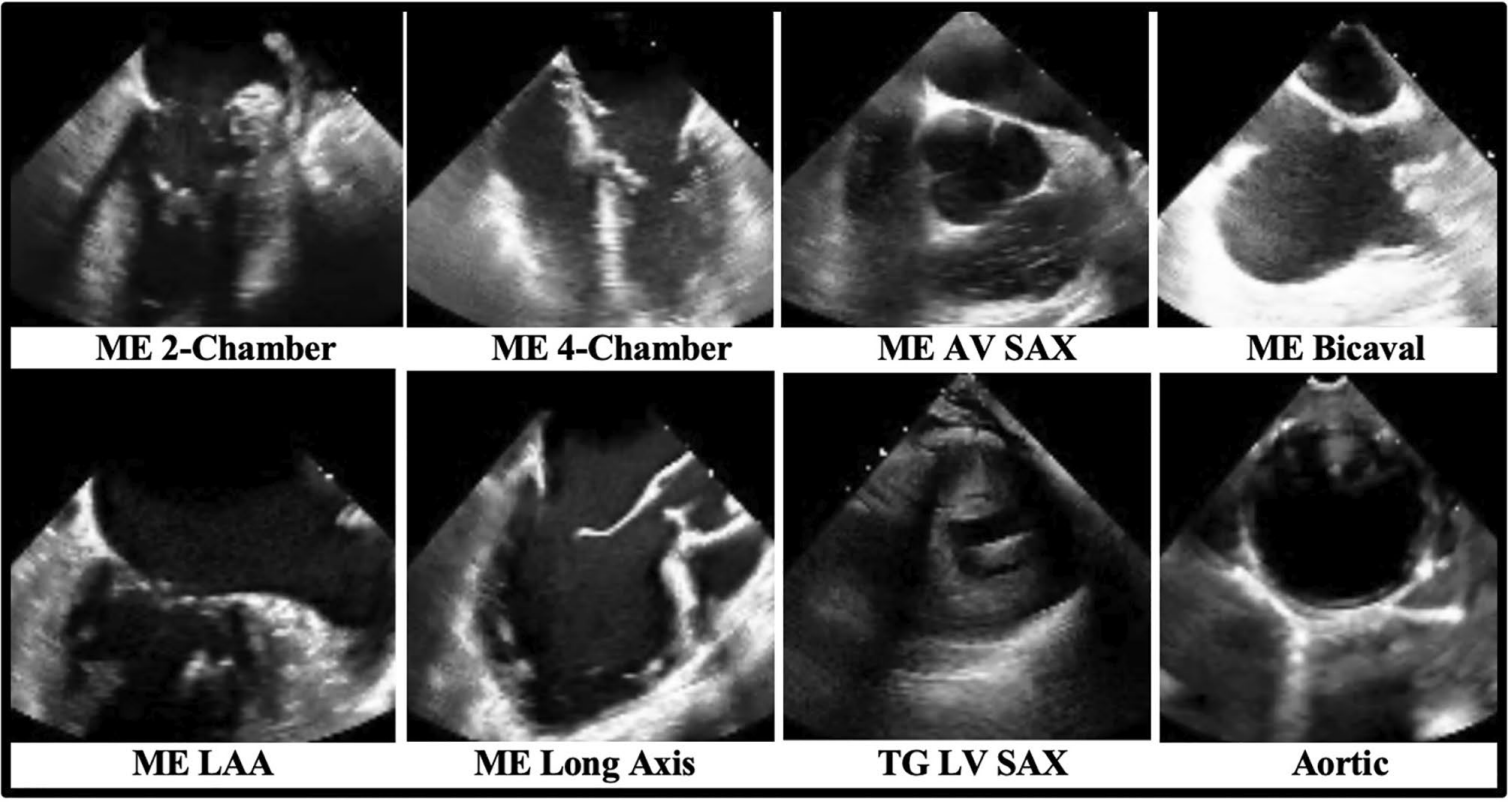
Sample training images used for the deep-learning view classification task. Images are 2-dimensional still frames sampled from the video data used in model training. Eight standard TEE views were chosen, including: the ME 2-Chamber View, ME 4-Chamber View, ME AV SAX View, ME Bicaval View, ME LAA View, ME Long Axis View, TG LV SAX View, and Aortic View. TEE, transesophageal echocardiography; ME, mid-esophageal; AV, aortic valve; SAX, short axis; LAA, left atrial appendage; TG, trans-gastric; LV, left ventricular.
